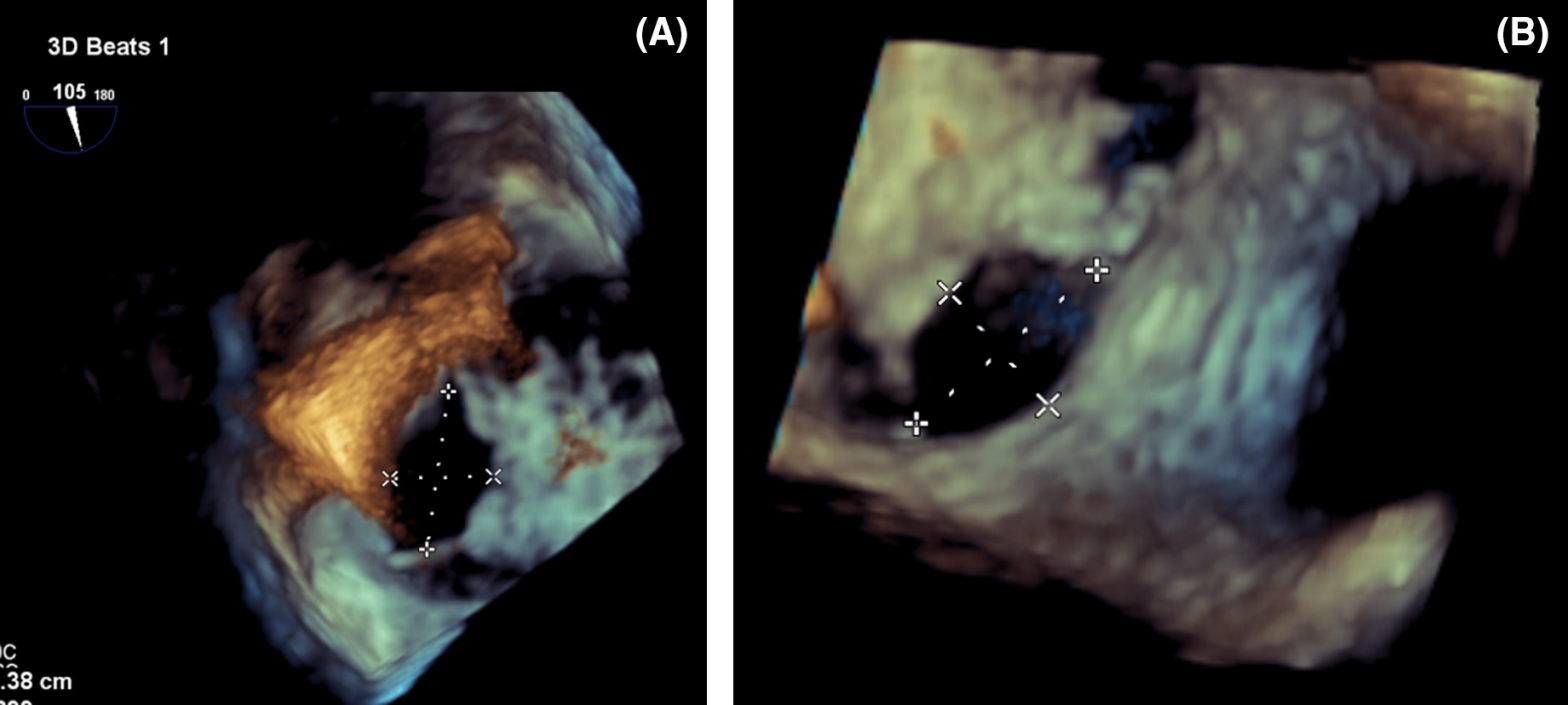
A,B: multiple ASD in 3D echocardiography
